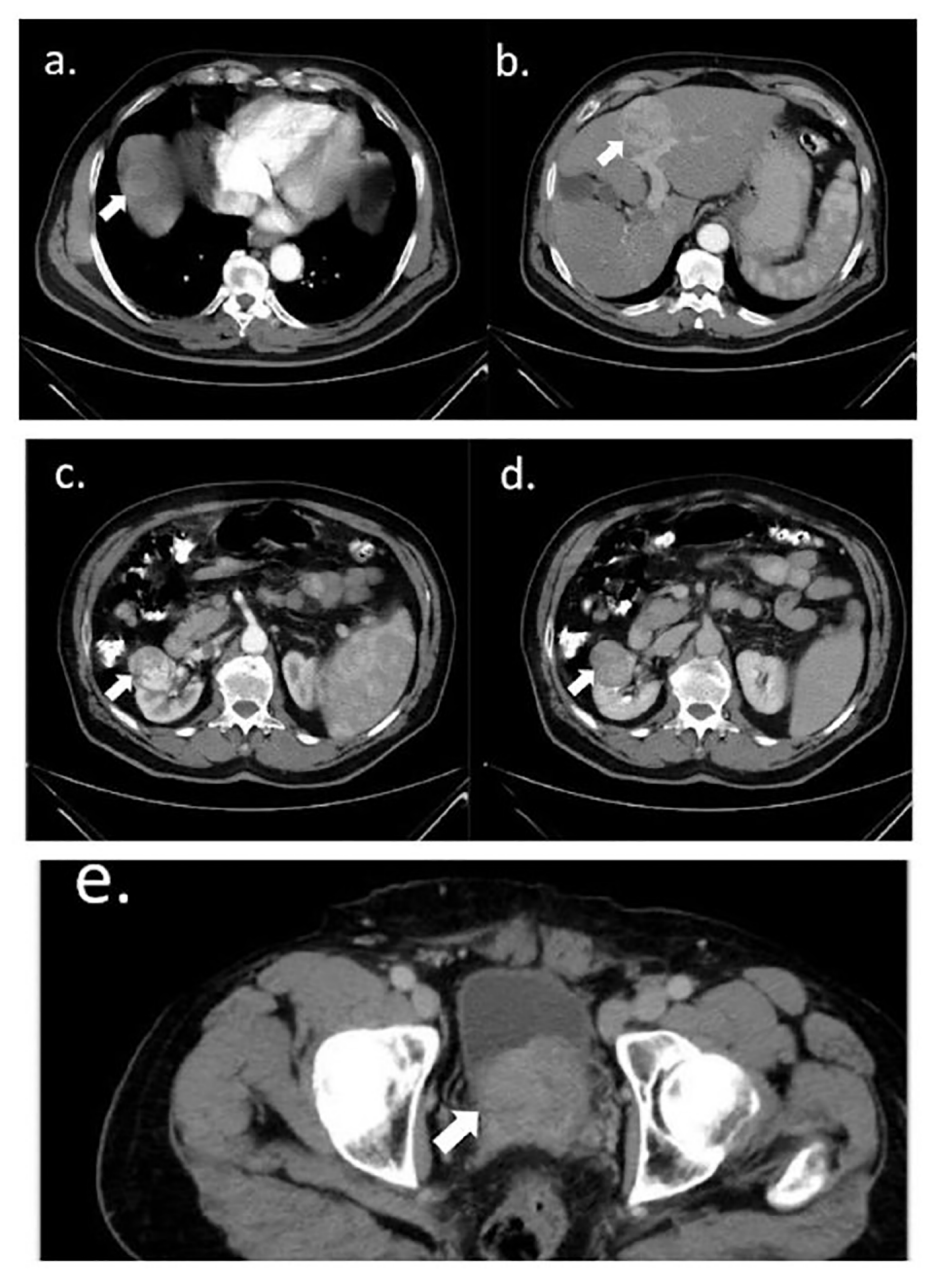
Abdomen and pelvis axial CT scan. (A, B) Hepatic isodense round lesions in the right lobe: a 5-cm lesion in segment four (A) and a 3.5-cm lesion in the dome of the liver (B), with heterogeneous enhancement in the portal phase. In addition to two smaller enhanced lesions in segment eight (B), the liver was normal in size and shape. (C, D) Right renal heterogeneously enhanced 4-cm lesion, arising from the right renal cortex, distorting adjacent mid-calyx and renal pelvis; (C) with and (D) without contrast. (E) An enlarged prostate of 6.3 cm projected to the base of the urinary bladder. All intended lesions are marked with arrows.
